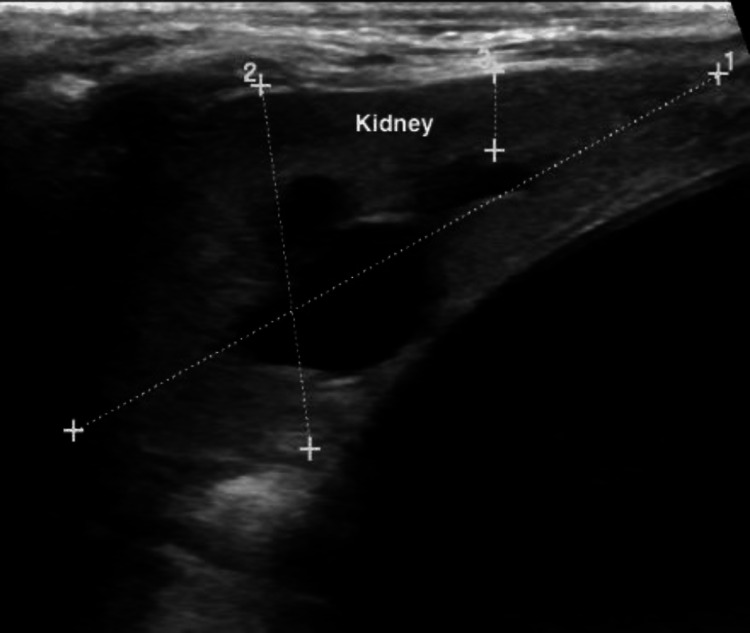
Ultrasound showing mild hydronephrosis.
